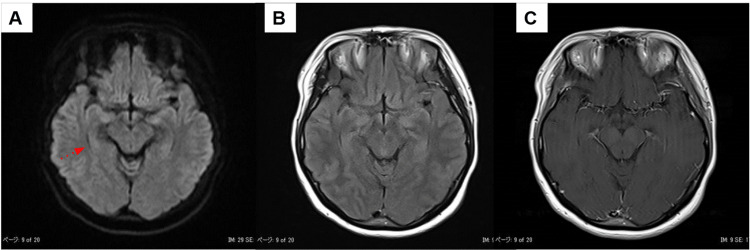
Brain MRI on admission (A) Axial DWI of the midbrain level. (B) Axial FLAIR image. (C) Axial T1-weighted image with gadolinium contrast. No intracranial hyperintense lesions or abnormal contrast enhancement were observed, including within the limbic system. DWI, Diffusion-weighted imaging; FLAIR, Fluid-attenuated inversion recovery; MRI, Magnetic resonance imaging
